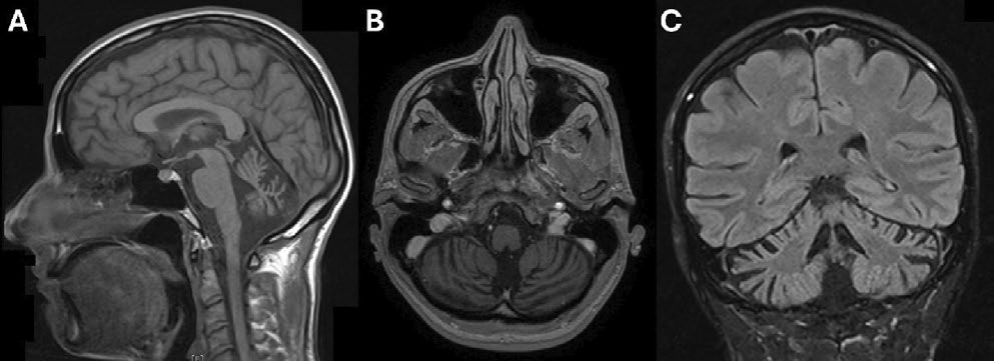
FIGURE 1 Brain MRI of the proband at 46 years old demonstrating cerebellum atrophy on T1‐weighted sagittal (A) and axial (B), and T2‐weighted coronal (C) sequences.
